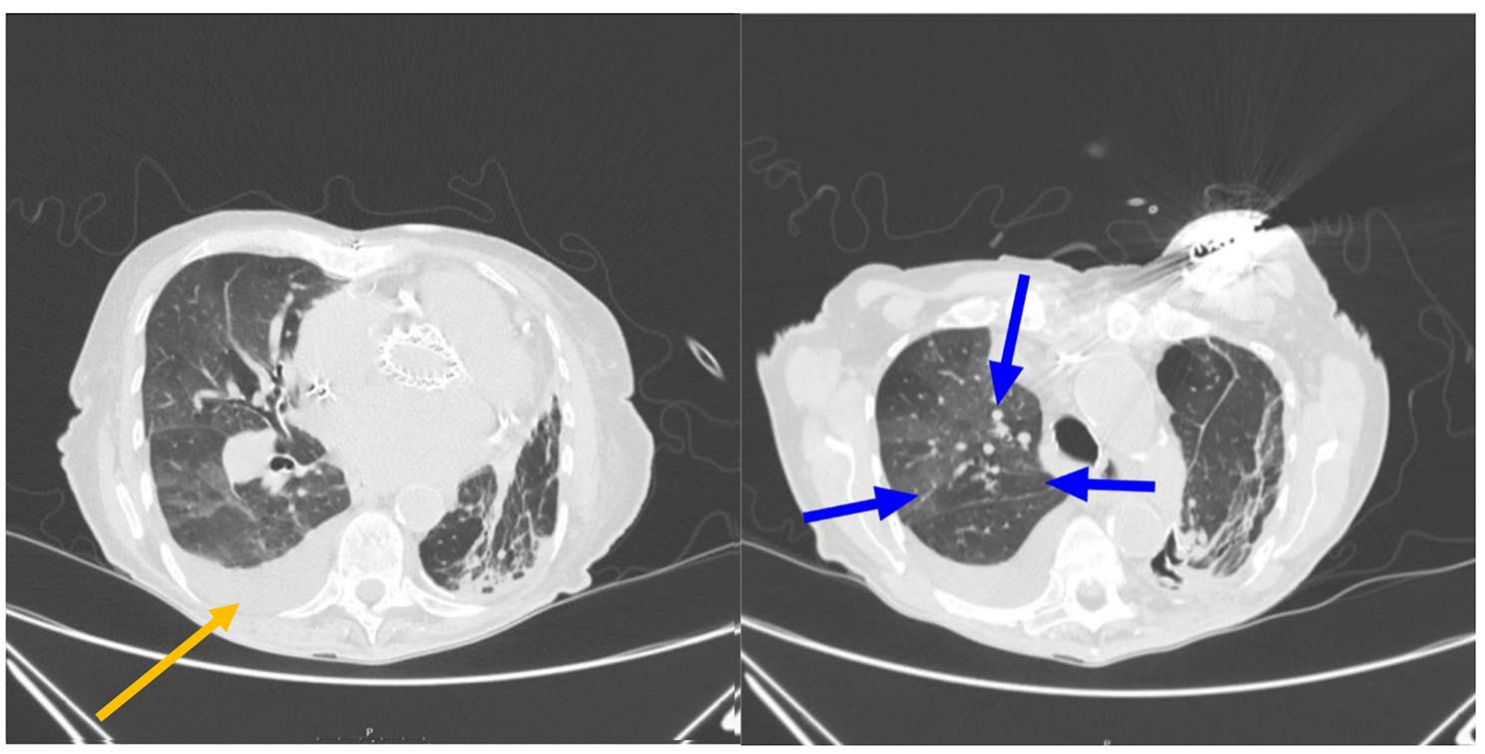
84 year old female with history of Monckeberg’s arteriosclerosis presenting with shortness of breath. Axial CT images in lung window shows (A) pleural effusion (orange arrow) and (B) interlobular septal thickening and ground glass opacities (blue arrows) representing pulmonary edema
